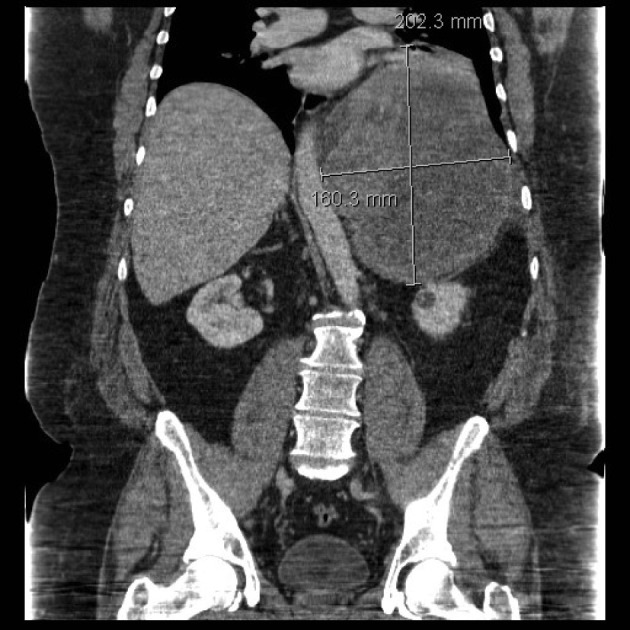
CT scan showing posterior mediastinal mass.
